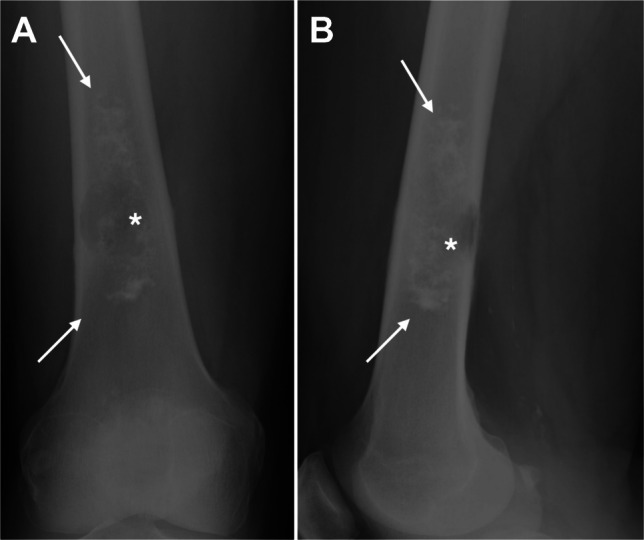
Initial [A] anteroposterior and [B] lateral radiographs of the distal femur demonstrating the biphasic lesion in the distal femoral shaft characterised by an elongated ovoid intramedullary mass with radiodense chondroid matrix mineralisation outlined at its craniocaudal margins (→), with central lytic component posteriorly (*) localised deep endosteal scalloping/periosteal reaction
